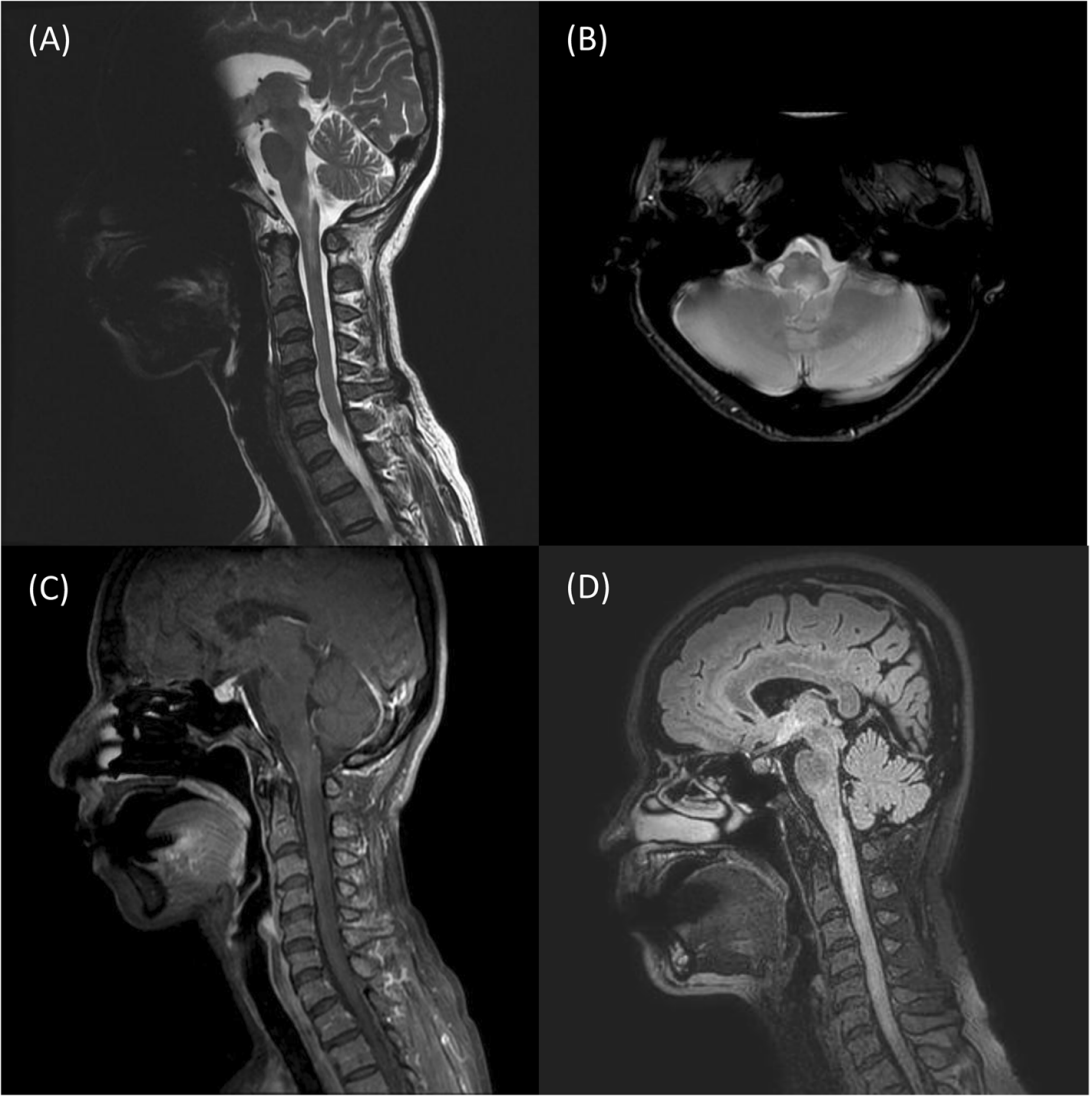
Brain MRI showed a representative case of a patient with NMOSD. a. A T2-weighted hyperintensity lesion at the area postrema that extended to the first cervical vertebral body level on the MRI sagittal view. b. The brain MRI axial view showed a hyperintense lesion at the area postrema that was more severe on the left side of the medulla. c. A [Gd]-enhanced lesion from the medulla to the first cervical vertebral body level on the sagittal-view T1-weighted contrast image. d. A hyperintense lesion from the area postrema extended to the secondary vertebral body level on the sagittal-view FLAIR image was noted 2 years after the first attack
